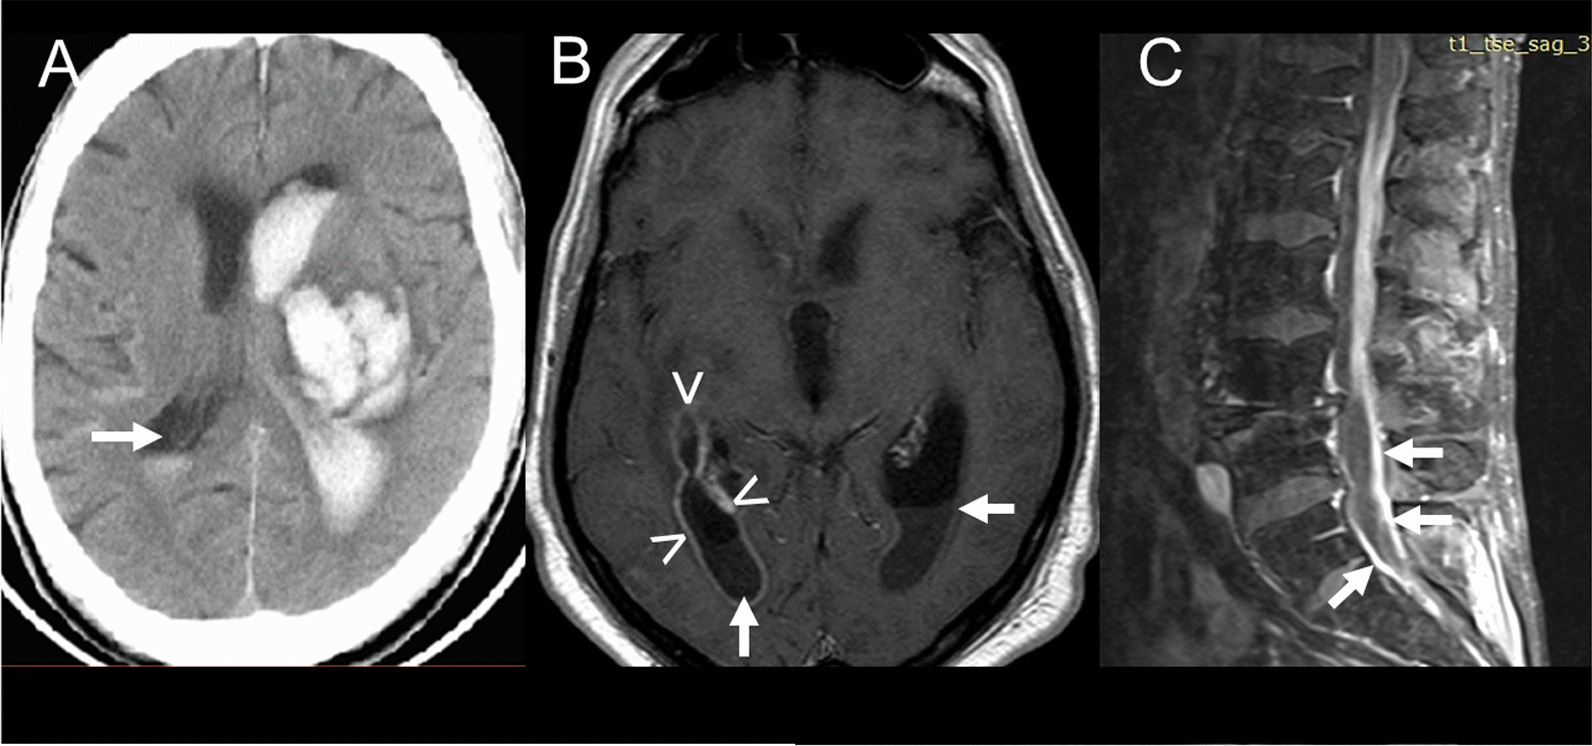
Sedimentation of cells in different parts of the ventricular system visible in clinical routine imaging. A Blood sedimentation in the 2nd ventricle (arrow) after intracerebral and intraventricular hemorrhage (cranial computer tomography) (kindly provided by Prof. Dr. Hilmar Prange, Dept. of Neurology, University Medicine Göttingen, Germany). B Pus in the dorsal horns of the 1st and 2nd ventricle (arrows), contrast enhancement of the wall of the right lateral ventricle (arrowheads) (Streptococcus intermedius meningitis and ventriculitis; T1-weighted magnetic resonance image plus gadolinium contrast enhancement). C Pus in the lumbar spinal canal (arrows) (*Candida albicans* meningitis; T1-weighted magnetic resonance image plus gadolinium contrast enhancement) (kindly provided by Dr. Hans-Heino Rustenbeck, Dept. of Neuroradiology, University Medicine Göttingen, Germany)
